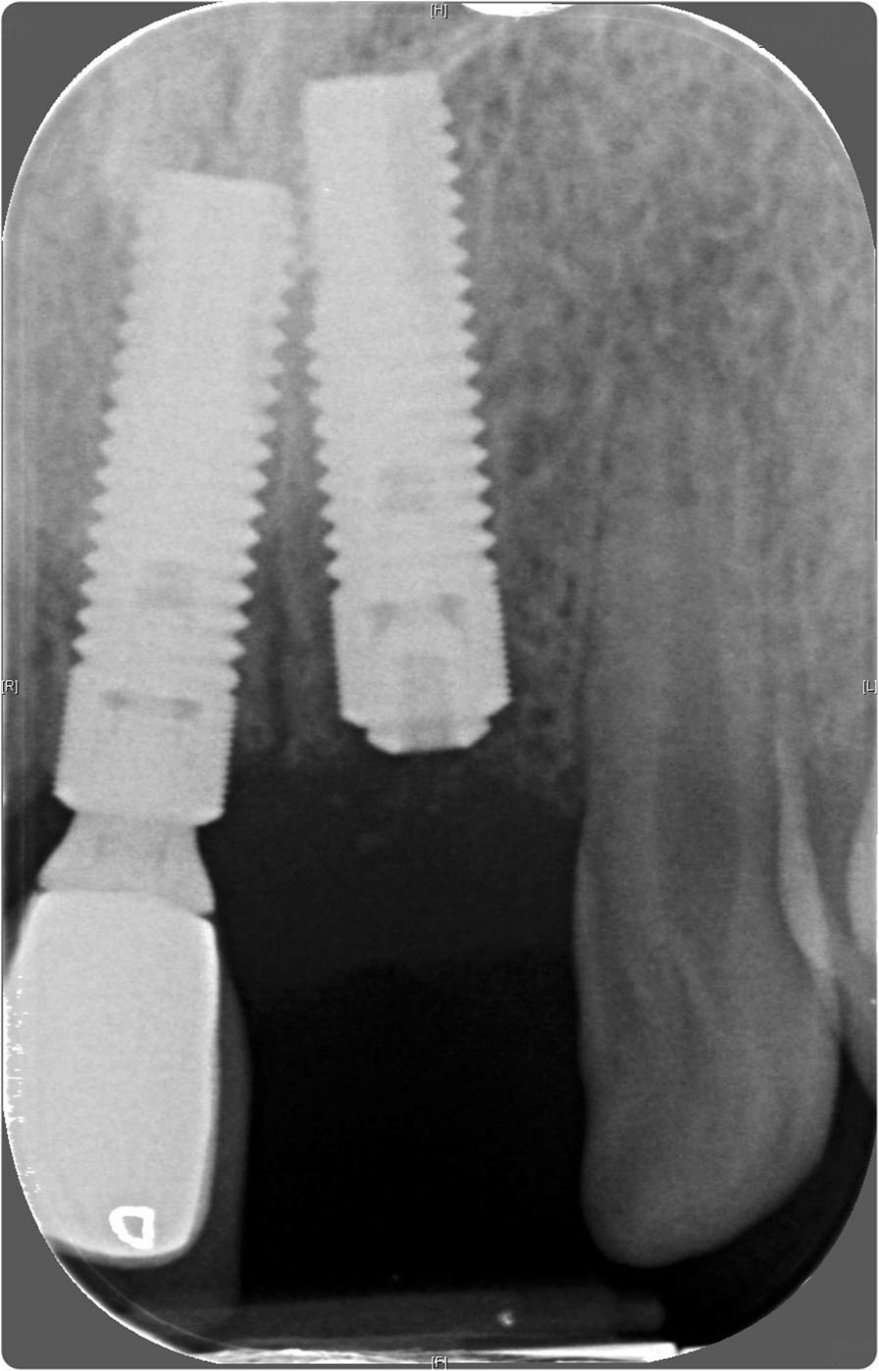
Placement confirmation. Radiograph of fixture in 21 position confirming angulation which is not ideal but acceptable
